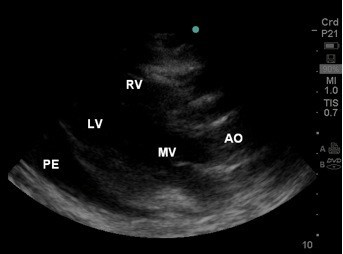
Emergency Department B-mode echocardiogram showing a large pericardial effusion (PE). LV, left ventricle; RV, right ventricle; MV, mitral valve; AO, aorta.
